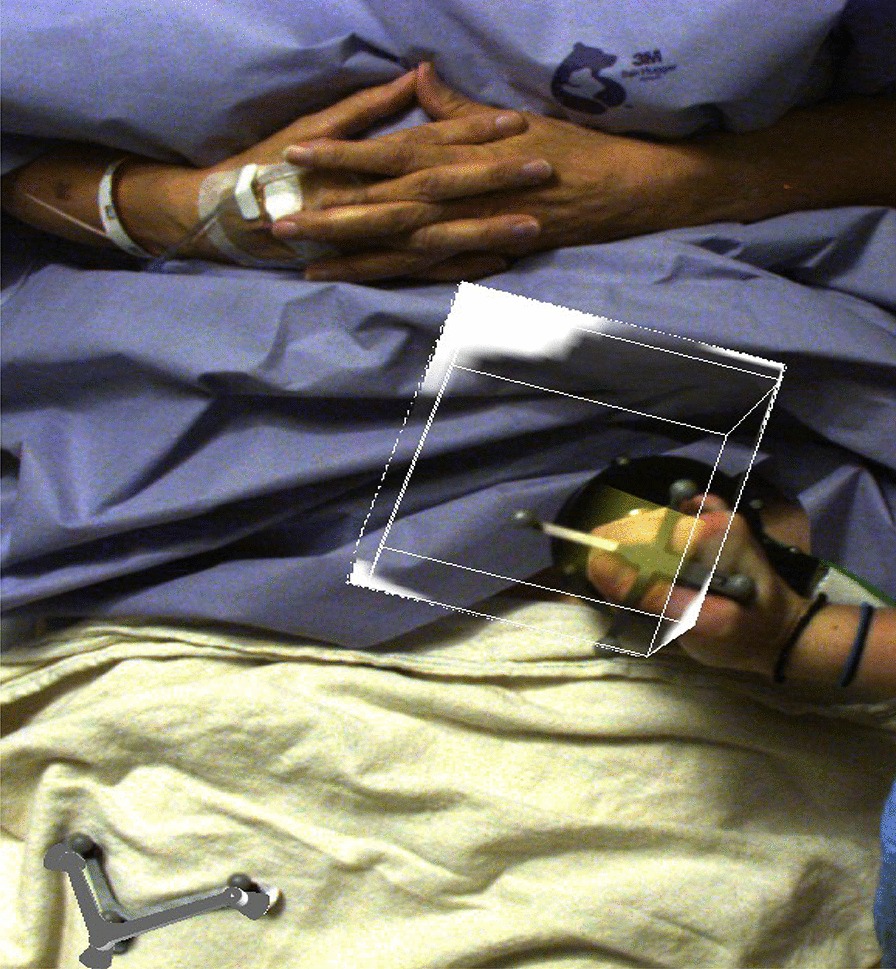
Subject being scanned with the camera fhSPECT system. The box indicates the volume that will be reconstructed
